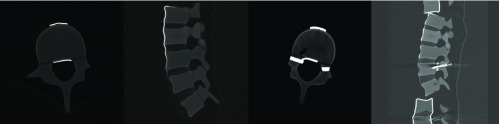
CT scanning of Sawbone spines: (from left to right) axial of control spine, sagittal control spine, axial image of distracted spine, sagittal image of distracted spine.
